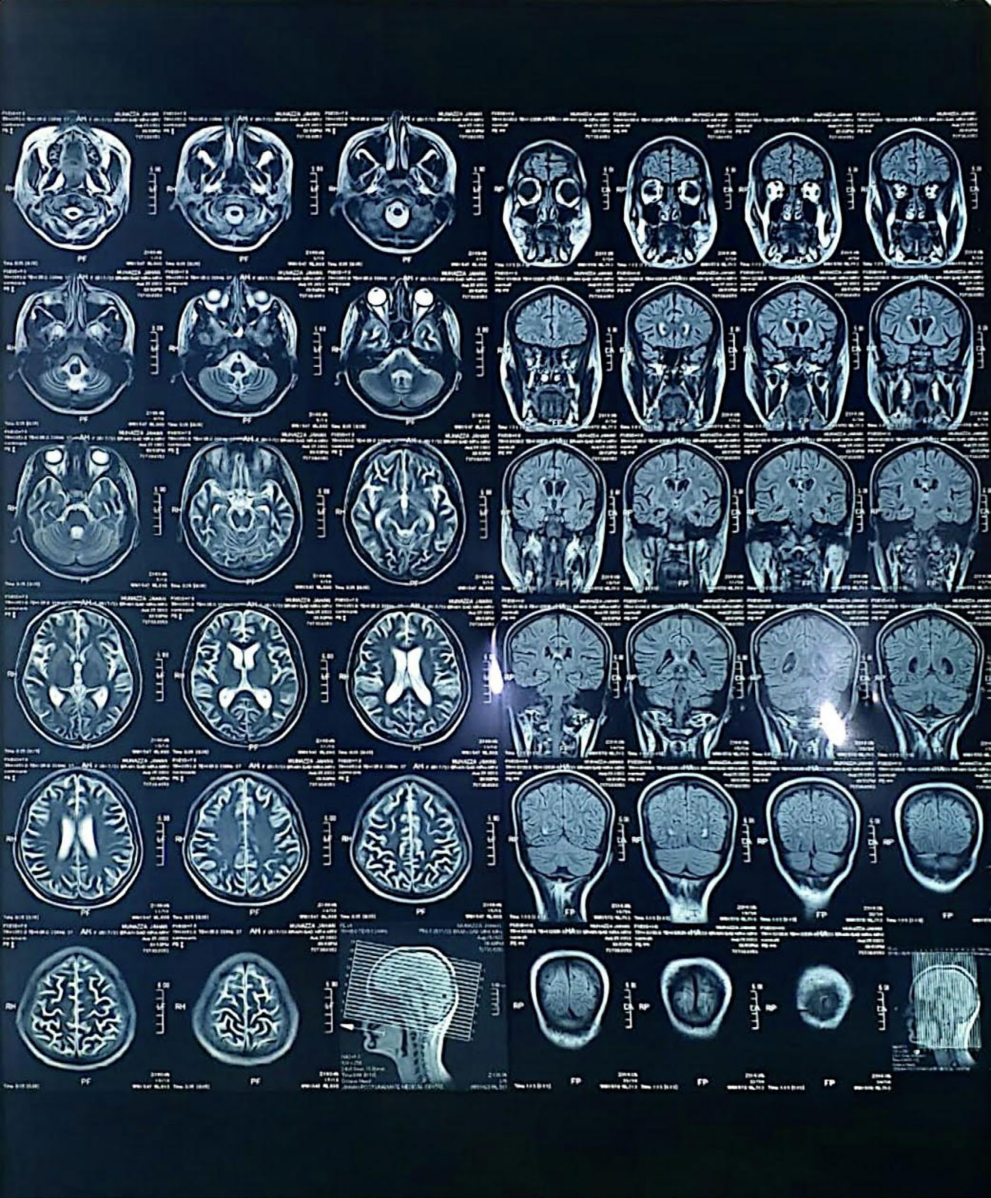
MRI Of the Brain Revealing A Dilated Ventricular System With Minimal Subependymal Seepage.
